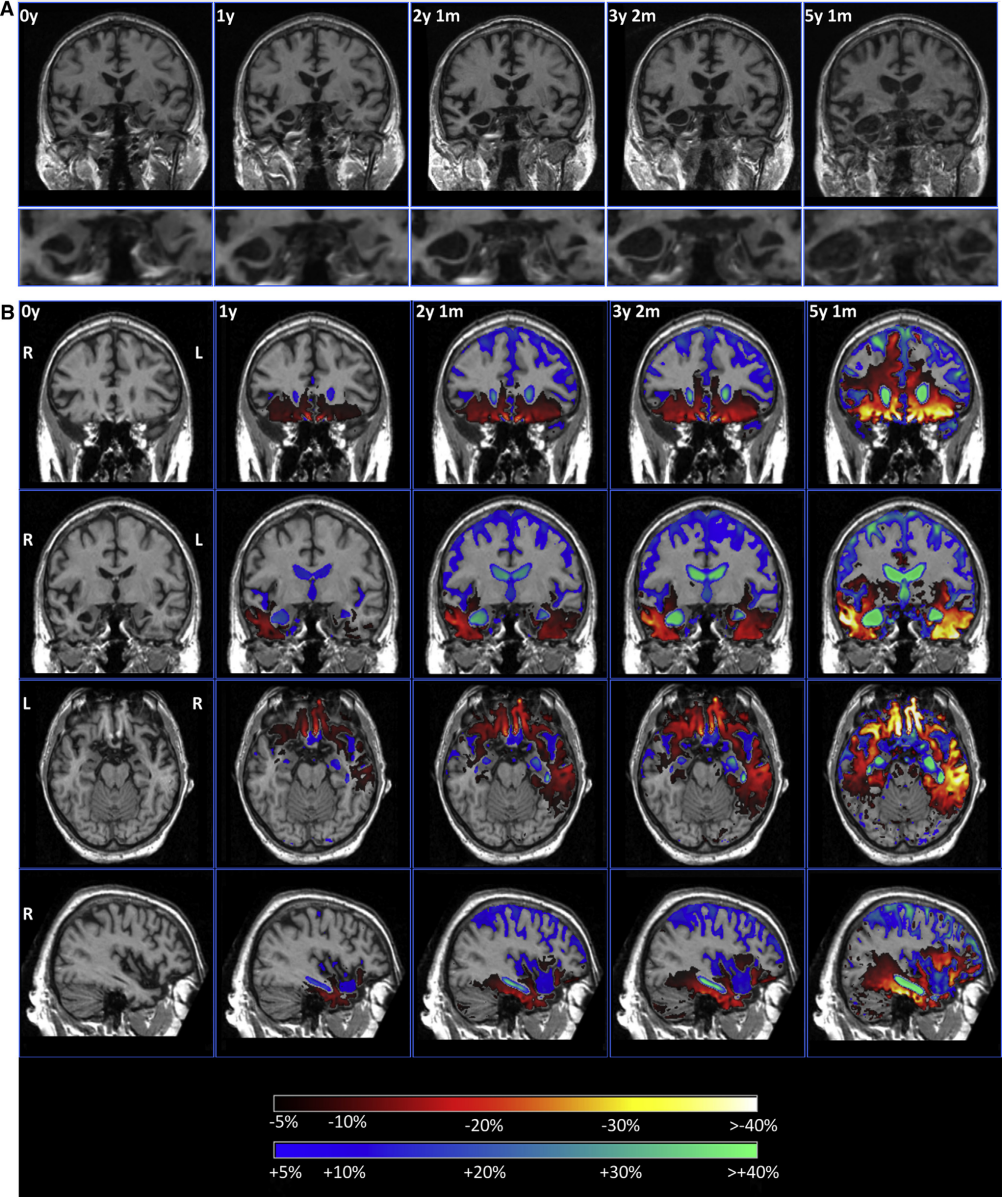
(A) Coronal sections of T1 volumetric magnetic resonance imaging scans at baseline and at the following four follow-up visits. In the lower panels, a close-up of the amygdala shows progressive asymmetrical medial temporal lobe atrophy. (B) Coronal sections at the level of the frontal (top) and mid-temporal lobes (second row), axial section through the orbitofrontal and medial temporal lobe (third row), and sagittal section through the right hemisphere (bottom). The first column represents the baseline scan with the four columns to the right showing a longitudinal SPM overlay (in comparison with the baseline scan) with yellow/red representing 5% or greater volumetric contraction and blue representing 5% or greater volumetric expansion. At the first follow-up scan atrophy is localized to the right temporal lobe and orbitofrontal lobe, but over time spreads dorsally and posteriorly, involving the frontal lobe, posterior temporal lobe, and similar areas in the contralateral hemisphere.
